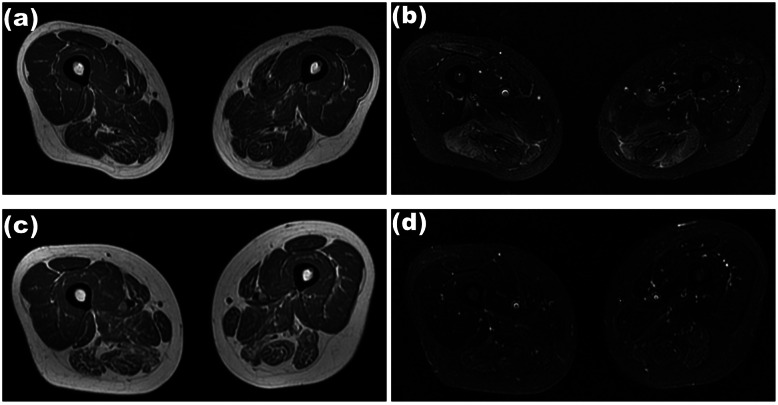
Thigh MRI of refractory ANM-SRP. Thigh MRI showed mild fatty infiltration (a; T1-weighted MRI) and focal edema (b; Short T1 inversion recovery sequences) in thigh muscle before treatment, the fatty infiltration aggravated (c) and muscle edema alleviated (d) after immunotherapy
